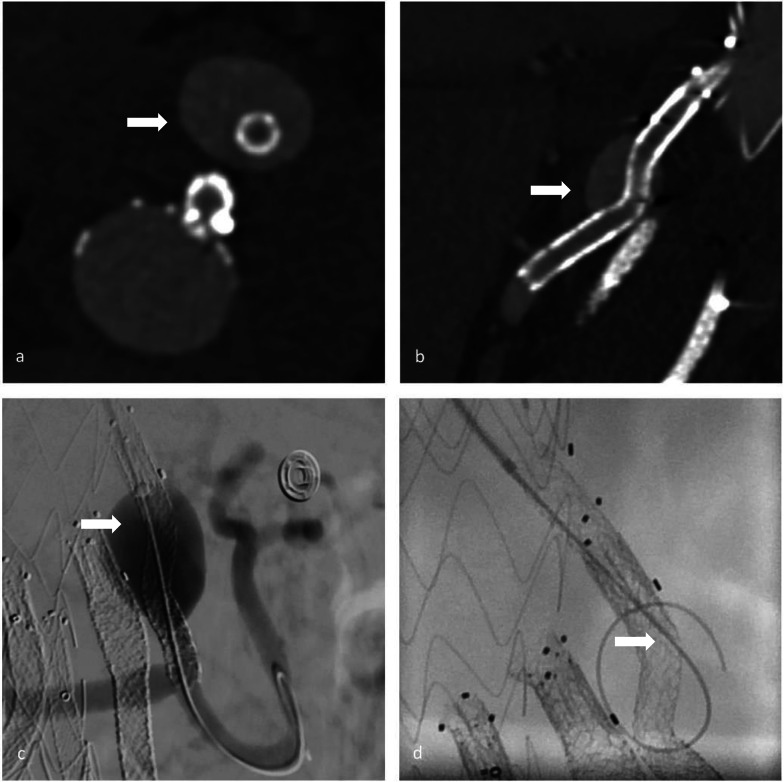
Membrane disruption and wire fracture of BSG (failure mode 2) in celiac trunk with an endoleak surrounding the BSG in CTA (a, b) and angiography (c). Guide wire passage was possible through the fractured BSG into the aneurysm (d)
